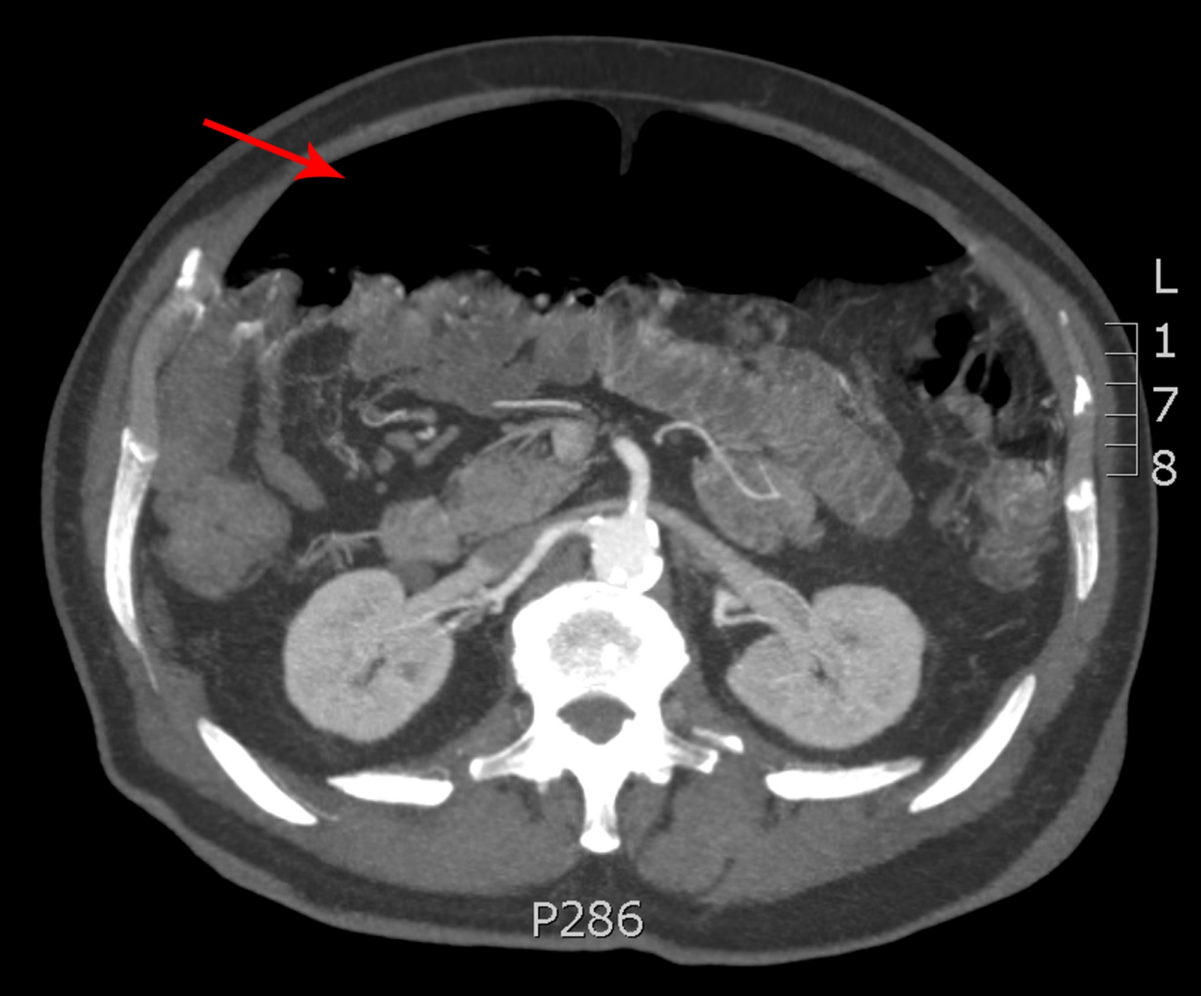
CT scan demonstrating extensive pneumoperitoneum (red arrow)
